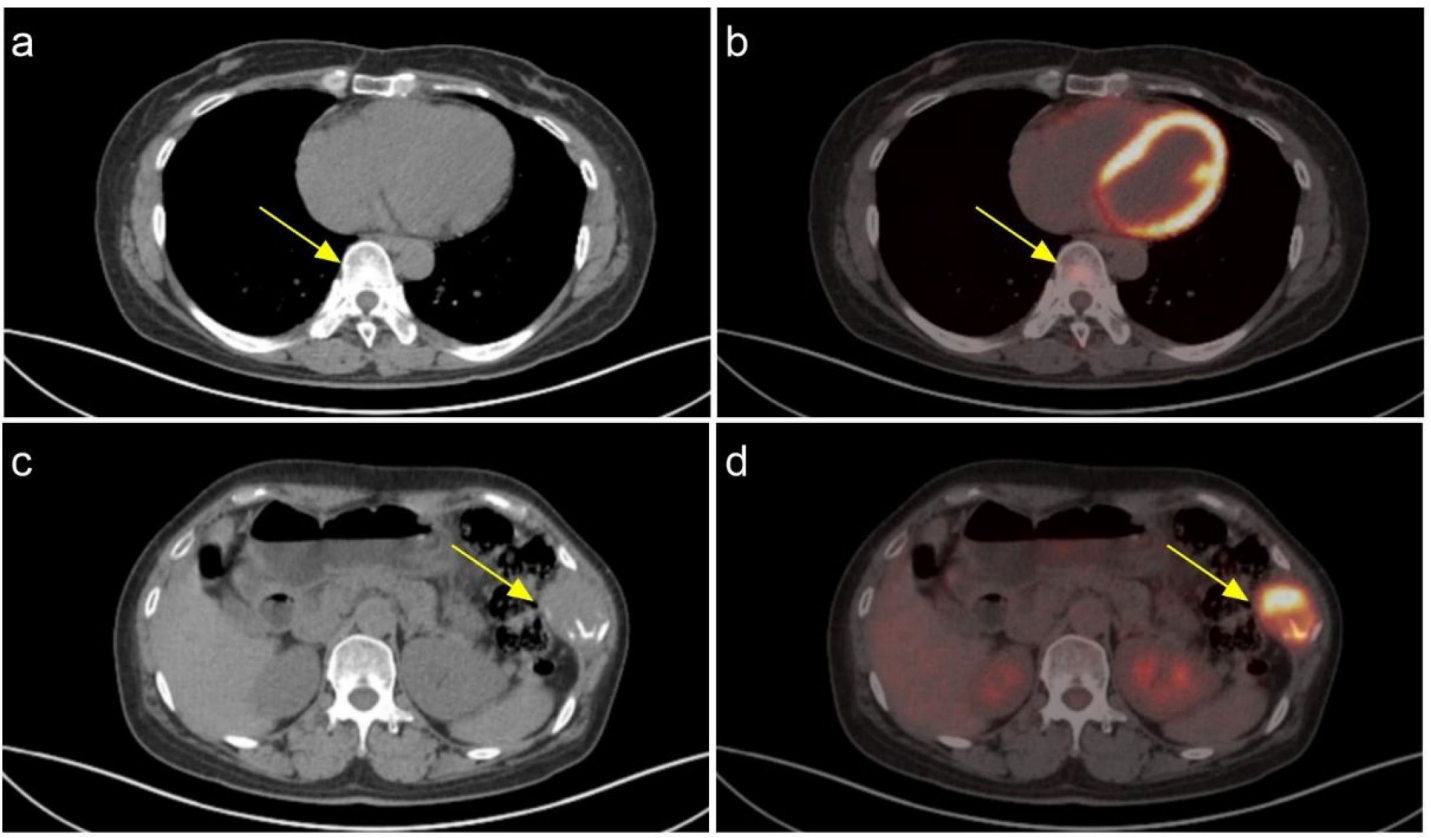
The increased glucose metabolism in the eighth thoracic vertebra is consistent with the imaging manifestations of a metastatic tumor. (A) CT (B) PET-CT; The increased glucose metabolism in the 9th rib on the left side is consistent with the radiological manifestations of a metastatic tumor. (C) CT (D) PET-CT.
